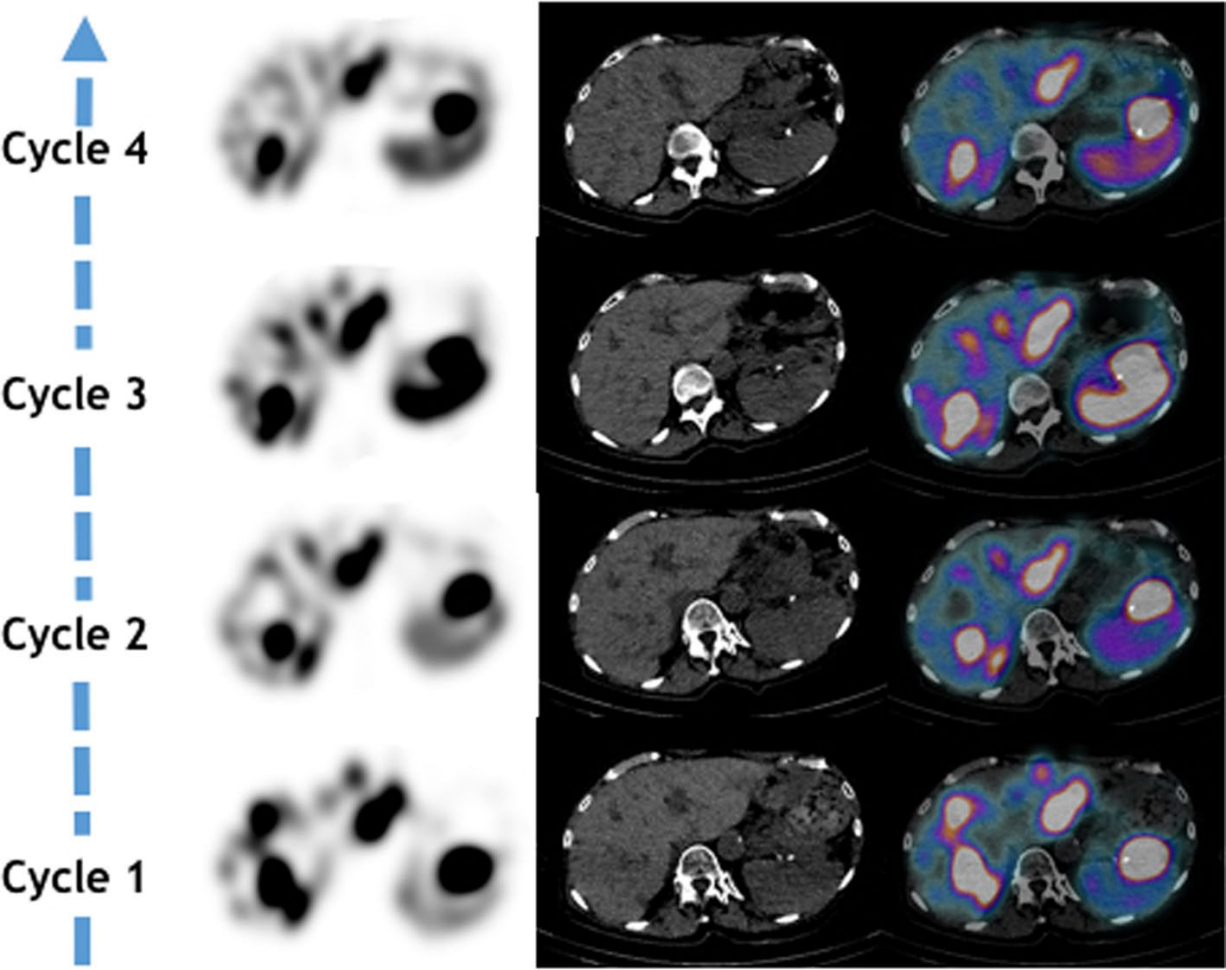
Axial slices of SPECT, CT and fused SPECT/CT images showing uptake in the primary pancreatic tumor and liver metastases after each cycle of PRRT
